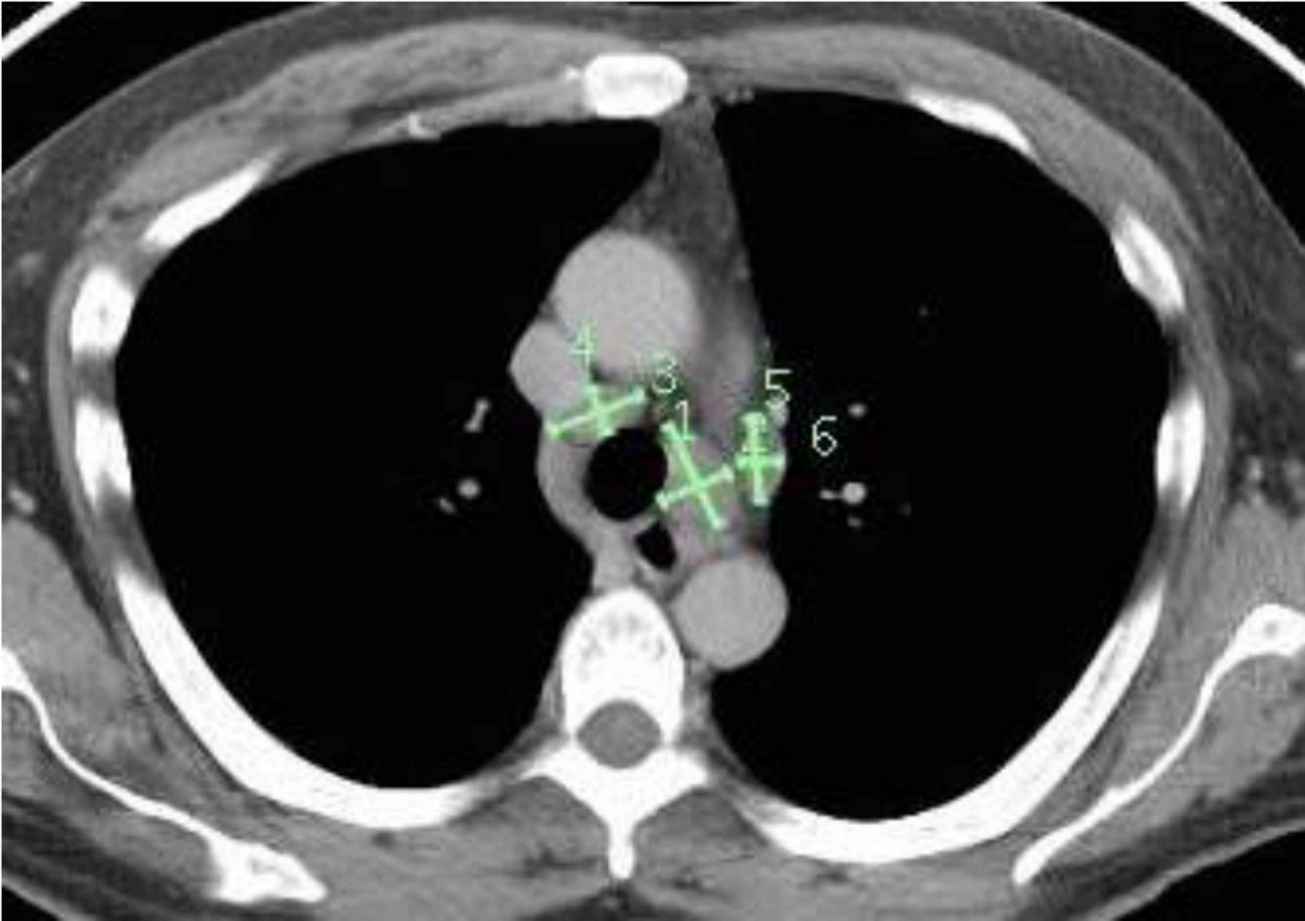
CT of the chest showing extensive mediastinal lymphadenopathy.
